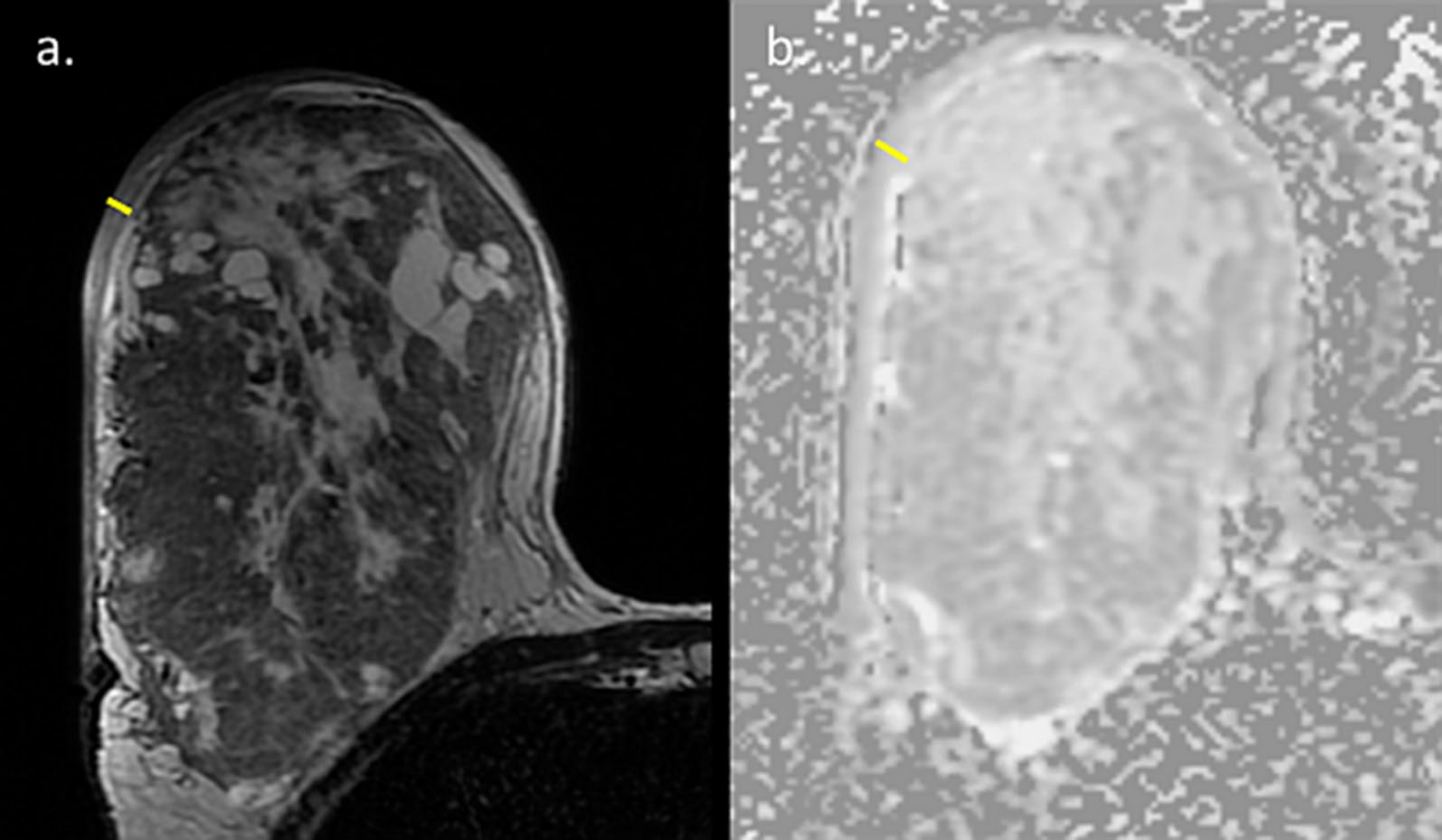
Mastitis. Unenhanced MR images of 37-year-old pregnant patient with refractory mastitis are presented. The patient presented with breast edema, erythema and pruritus and blinded subcutaneous punch biopsies revealed adenosis on pathology. Because of continuous symptoms despite treatment, we were requested to perform MRI without contrast injection to rule out underlying inflammatory carcinoma. T2 weighted image revealed thickened skin (a) (yellow line), while no focal restriction was noted on ADC map (b)
